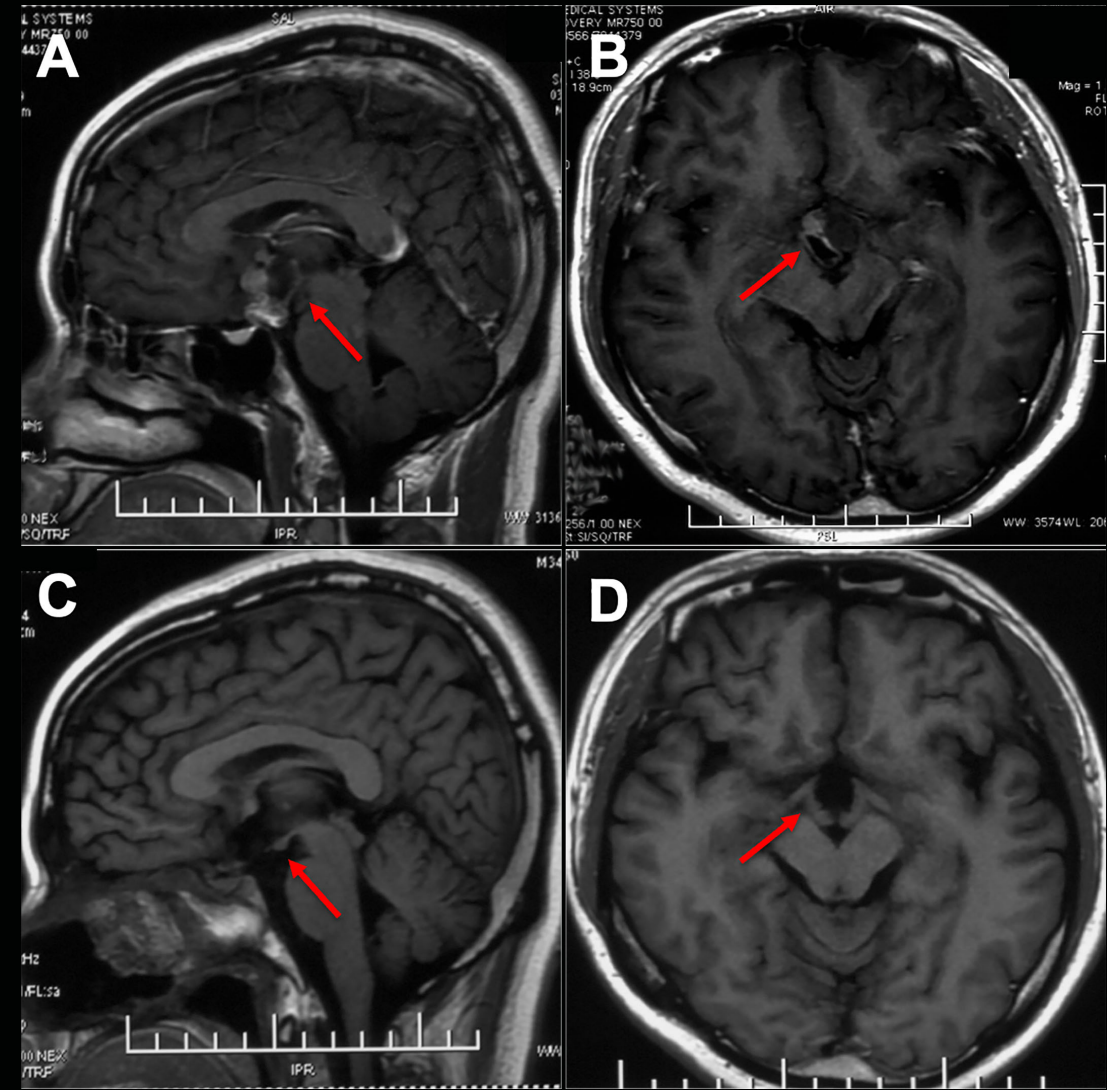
Preoperative and postoperative imaging of ITVC and intact mammary body. (A, B) There was a cystic and solid lesion on the suprasellar region. (C, D) The optic tract, third ventricle floor and mammillary bodies were well protected postoperatively on MR images.
